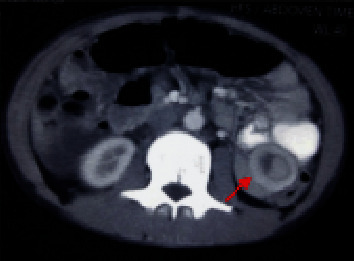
Computed tomography scan demonstrates jejunojejunal intussusception with a target sign (arrow) and minimal dilated proximal jejunal loop.
